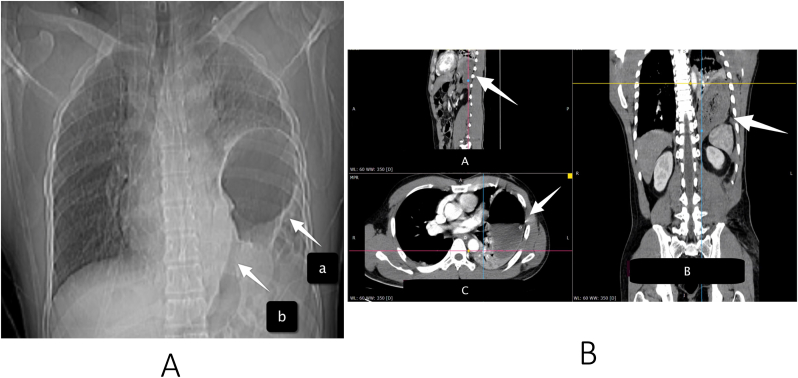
Left: Patient's initial chest x-ray, air sac (a) in the left thorax associated with the abdominal cavity (b); Right: A) Abdominal CT Scan-sagittal view B) coronal view C) axial view. The arrow shows the hollow viscus organ entering the thoracic cavity.
